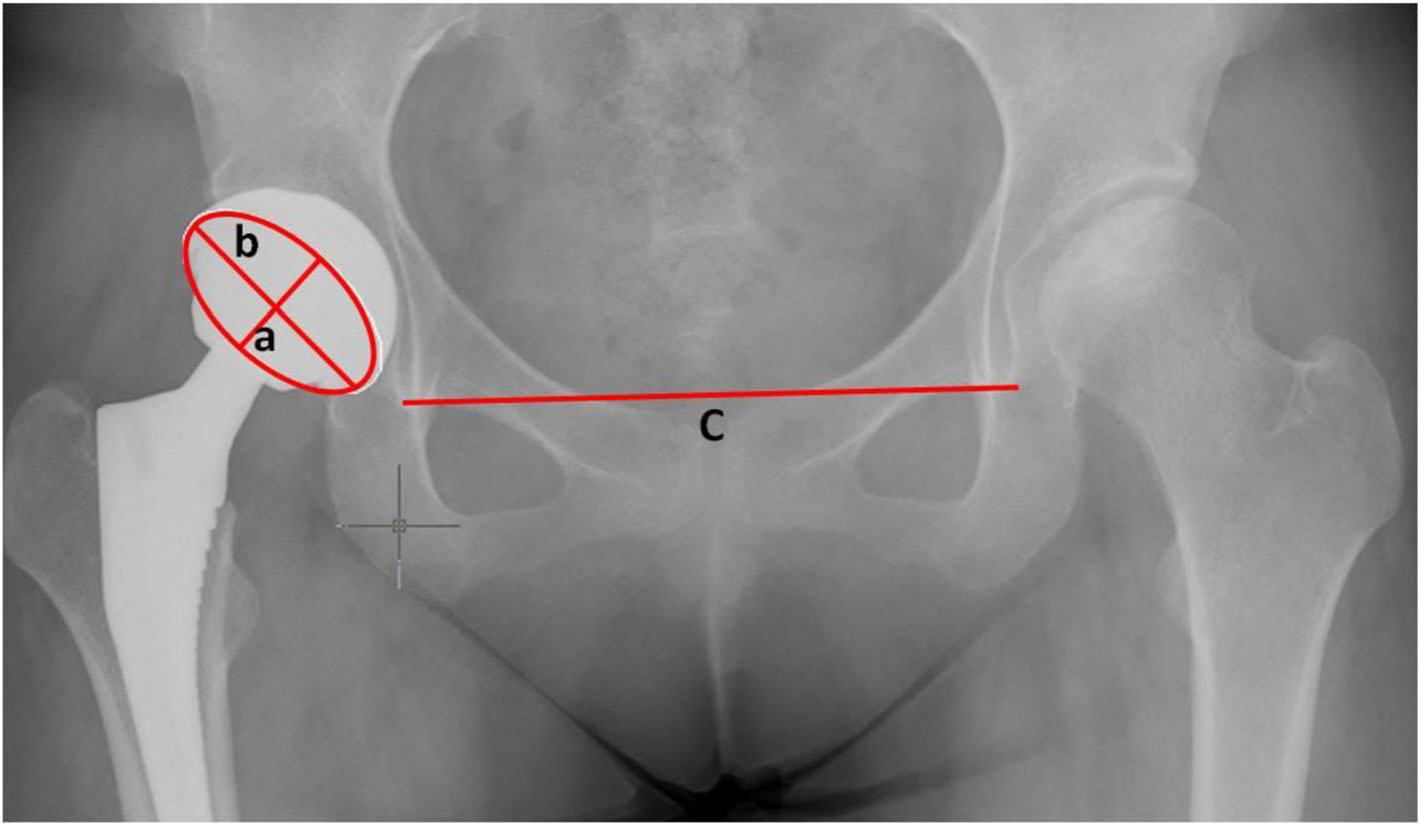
Measurements of the angles of inclination and anteversion on standing anteroposterior pelvic radiographs. The angle of inclination is the angle between the large diameter of the opening ellipse (b) and the interteardrop line (c). The angle of anteversion is the arcsine of the ratio of the small (a) and the large radius (b) of the opening ellipse, (= arcsin(a/b))
